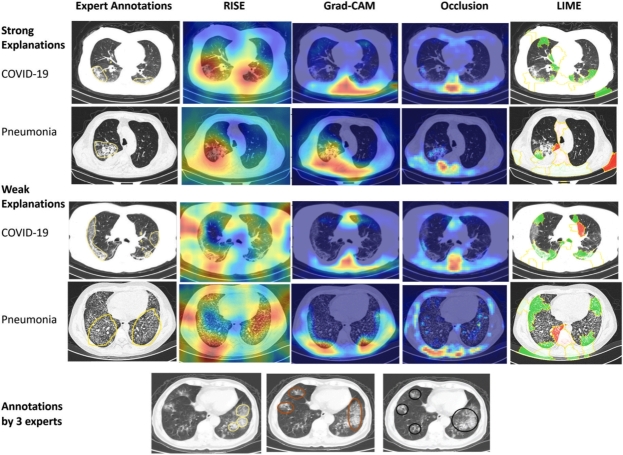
RISE, Grad-CAM, OA and LIME explanations by [65], display human annotations and explanations generated by mentioned methods for a COVID-19 CT image. Each explanation technique highlights salient regions responsible for the prediction. Human annotations highlight different salient regions. In the generated explanations, red regions indicate areas contributing to the prediction when using RISE, Grad-CAM, and OA. LIME differentiates pixels supporting the prediction in green and those negating the prediction in red.
